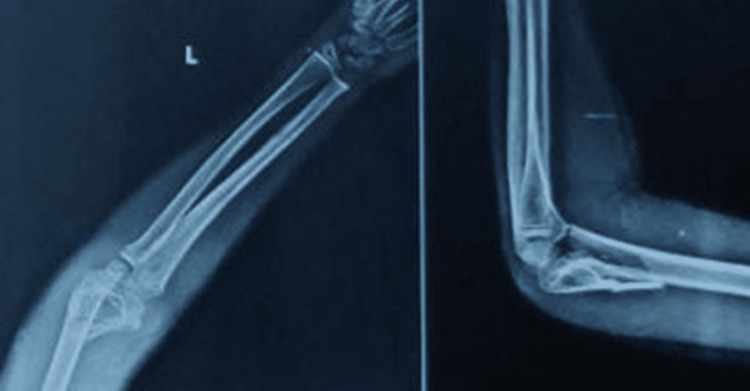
Radiograph of the left elbow. Anteroposterior and mediolateral views, suggestive of a comminuted displaced supracondylar humerus fracture.
